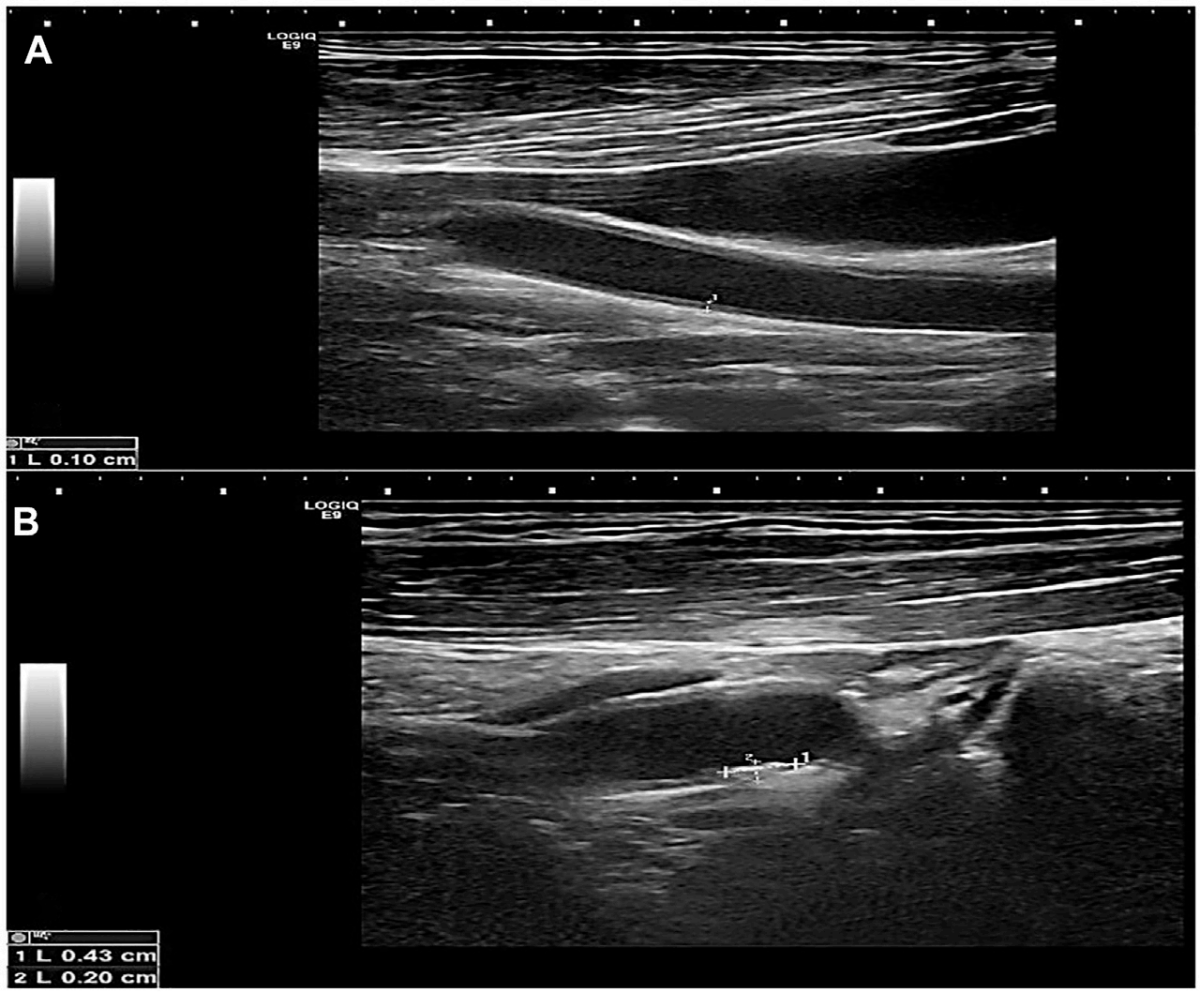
Greyscale longitudinal ultrasound images in a 60-year-old male diabetic patient showing (A) increased intimal/medial thickness at the left common carotid artery and (B) a calcified plaque at the proximal aspect of the left internal carotid artery.
